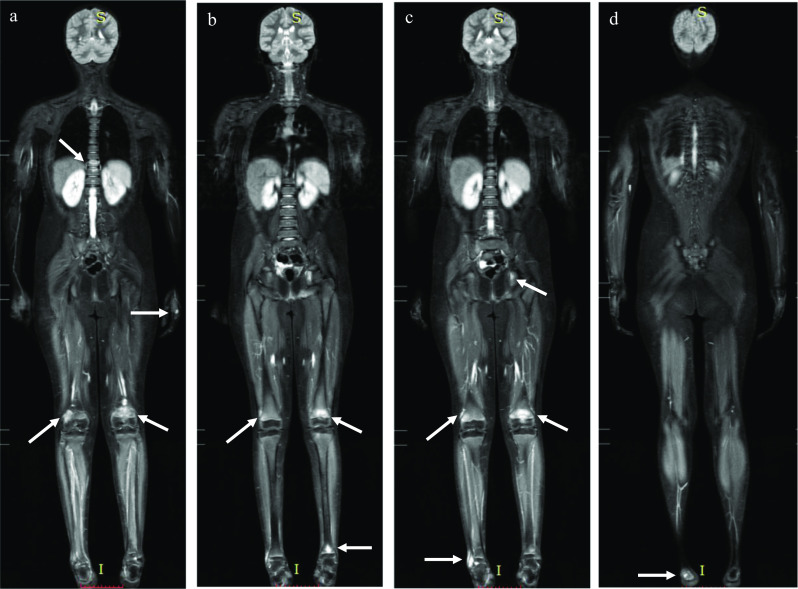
CNO in an 8-year-old girl complaining of hip and leg pain. Whole-body MRI (a–d) depicted multifocal bone lesions at the distal metaphysis of both femurs (a–c), distal metaphysis of the left tibia (b) and right fibula (c), right calcaneus (d), left acetabulum (c), left metacarpal bone head (a) and bone marrow edema at a mid-thoracic vertebral body (a). The vertebral lesion was asymptomatic and its detection in a preclinical scenario may change the treatment approach and prevent complications
